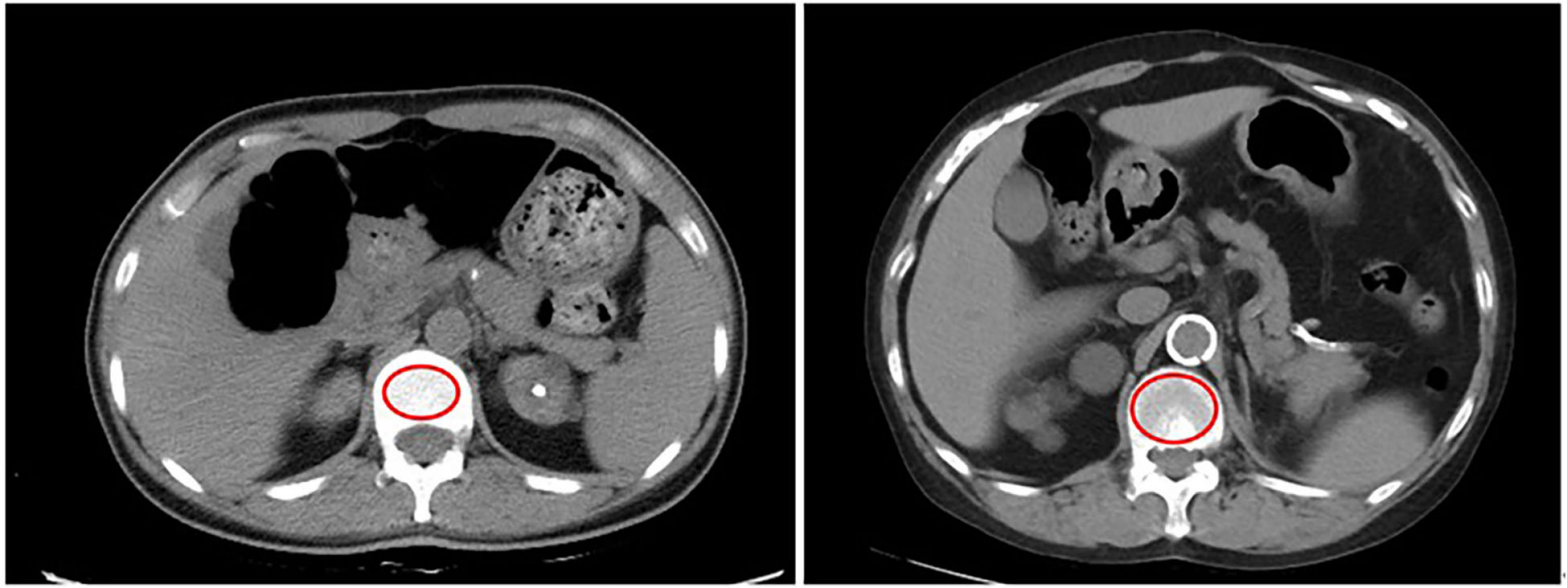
Axial image through the vertebral body of L1 on a thoracoabdominal-pelvic CT scan. Placement of the region of interest within the trabecular bone and assessment of the CT attenuation value in Hounsfield units (182.3 HU left and 96.5 HU right in this example)
